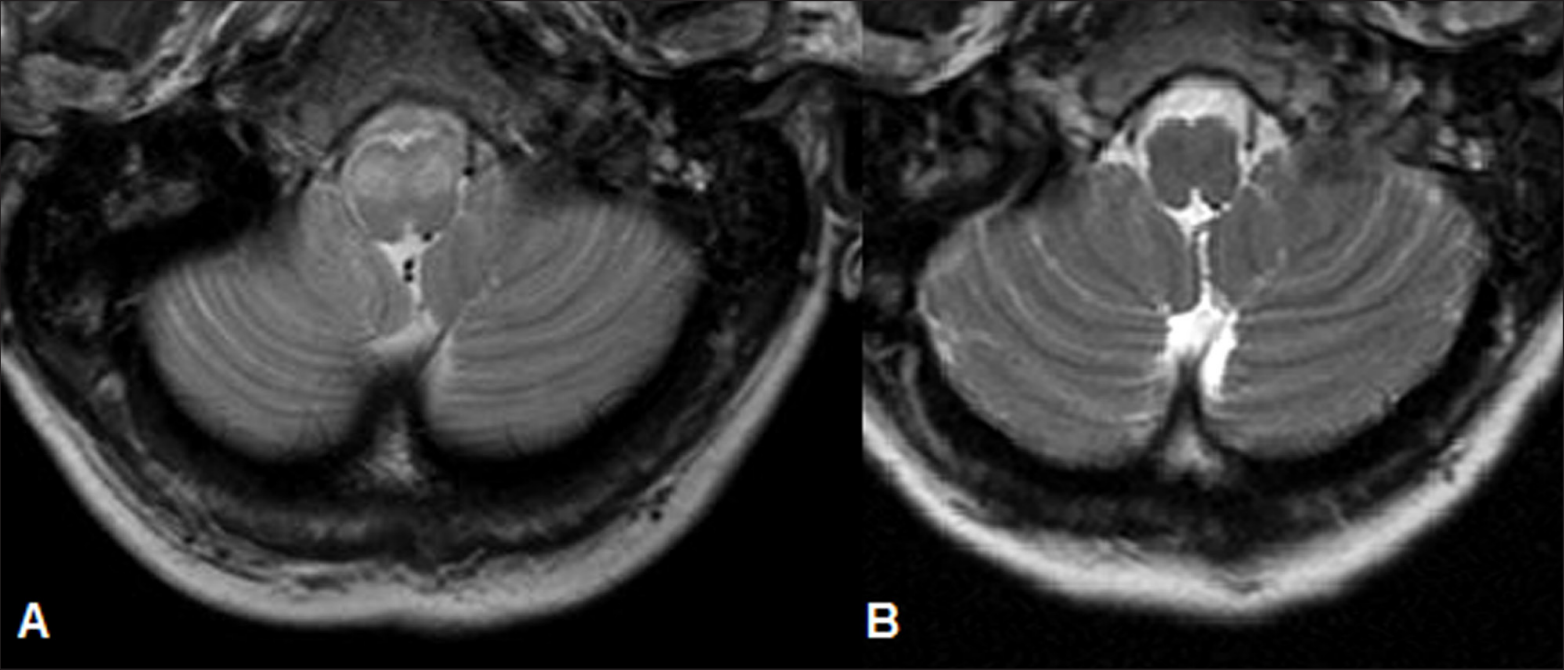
Axial T2-weighted image at the level of the medulla shows symmetric hyperintensity and expansion of the medullary olives (A). Axial T2-weighted image at the same level in the same patient but eight years earlier showed no abnormality (B).
